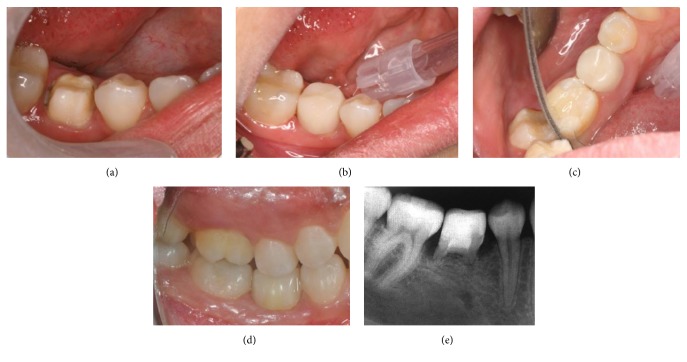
Crown preparation and cementation. (a) Tooth preparation with chamfered margin. (b–d) Intraoral views of the crown after cementation. (e) Postoperative radiograph of the crown.
